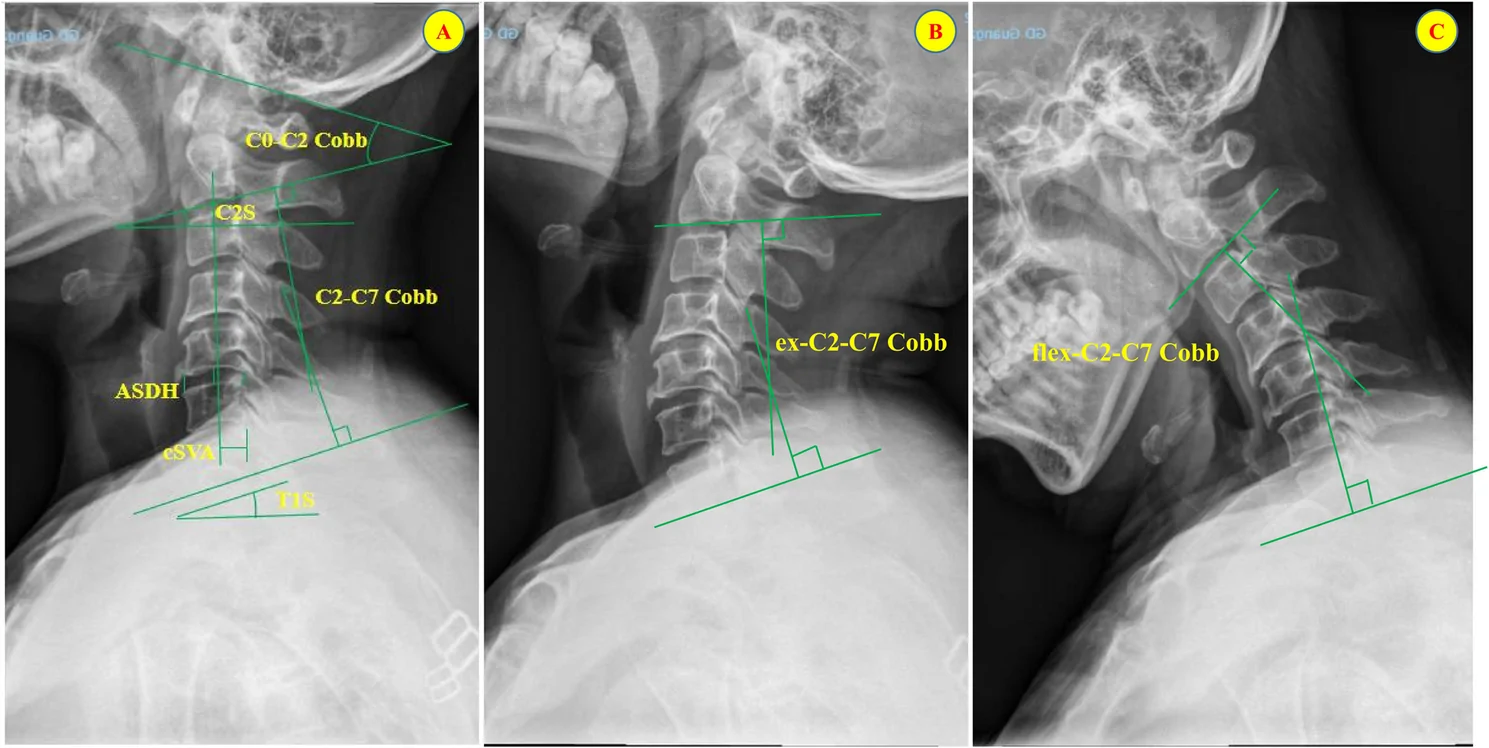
Measurement of cervical sagittal parameters. A: Neutral lateral radiograph demonstrating the C2–C7 Cobb angle, C0–C2 Cobb angle, cSVA, ASDH, T1 slope, and C2 slope; B:Hyperextension view showing the C2–C7 Cobb angle; C: Hyperflexion view showing the C2–C7 Cobb angle
